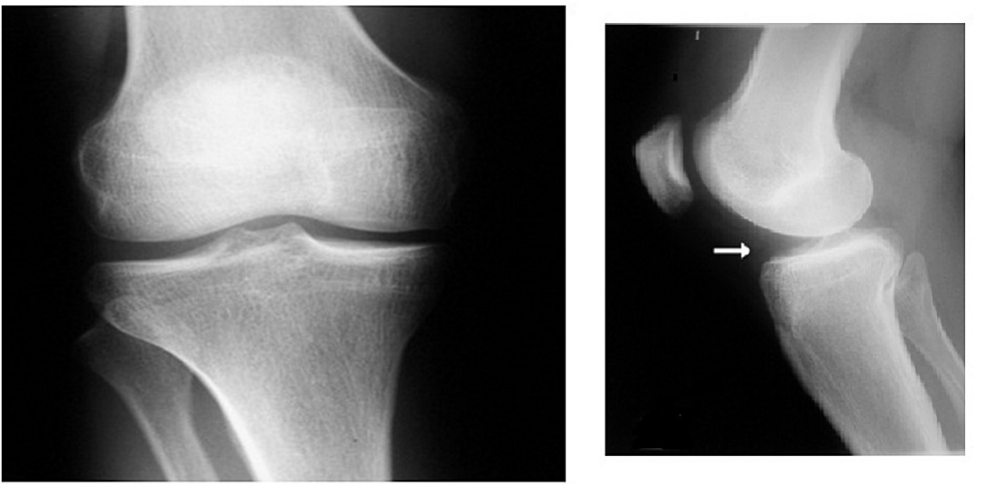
Plain radiography films of the knee in anteroposterior and lateral view showing no abnormality
